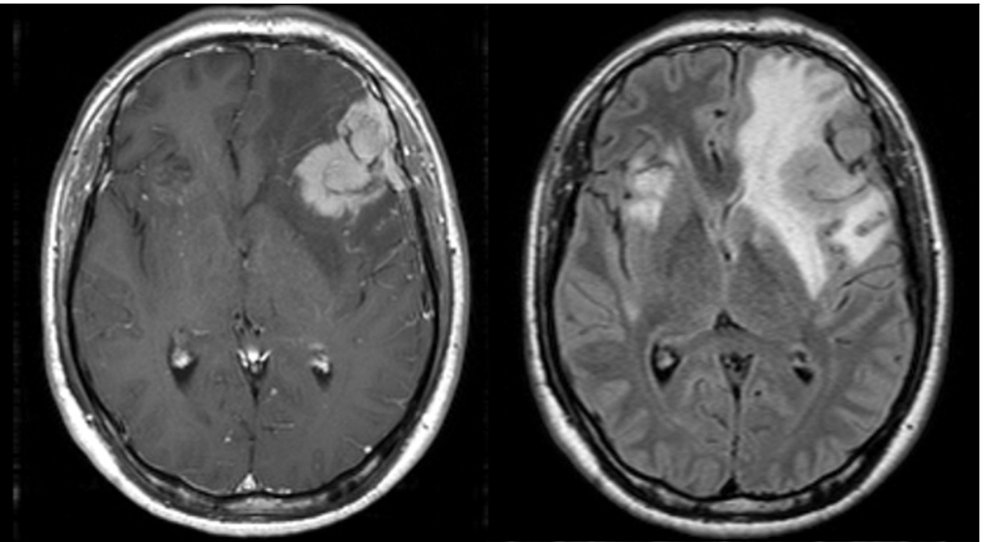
Concerns with multiple brain metastases. T1 post-contrast MRI with two left frontal brain metastases in close proximity (left) with significant associated edema after treatment on T2 FLAIR MR (right). MRI = magnetic resonance imaging; FLAIR = fluid attenuated inversion recovery
